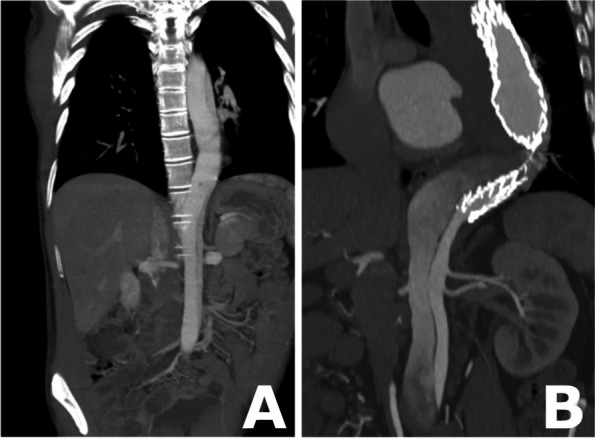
A Coronal CT image of the descending aorta of the patient after the surgery in 2013. B Coronal CT image of the descending aorta of the patient when the decision to apply the CP was made in 2021
